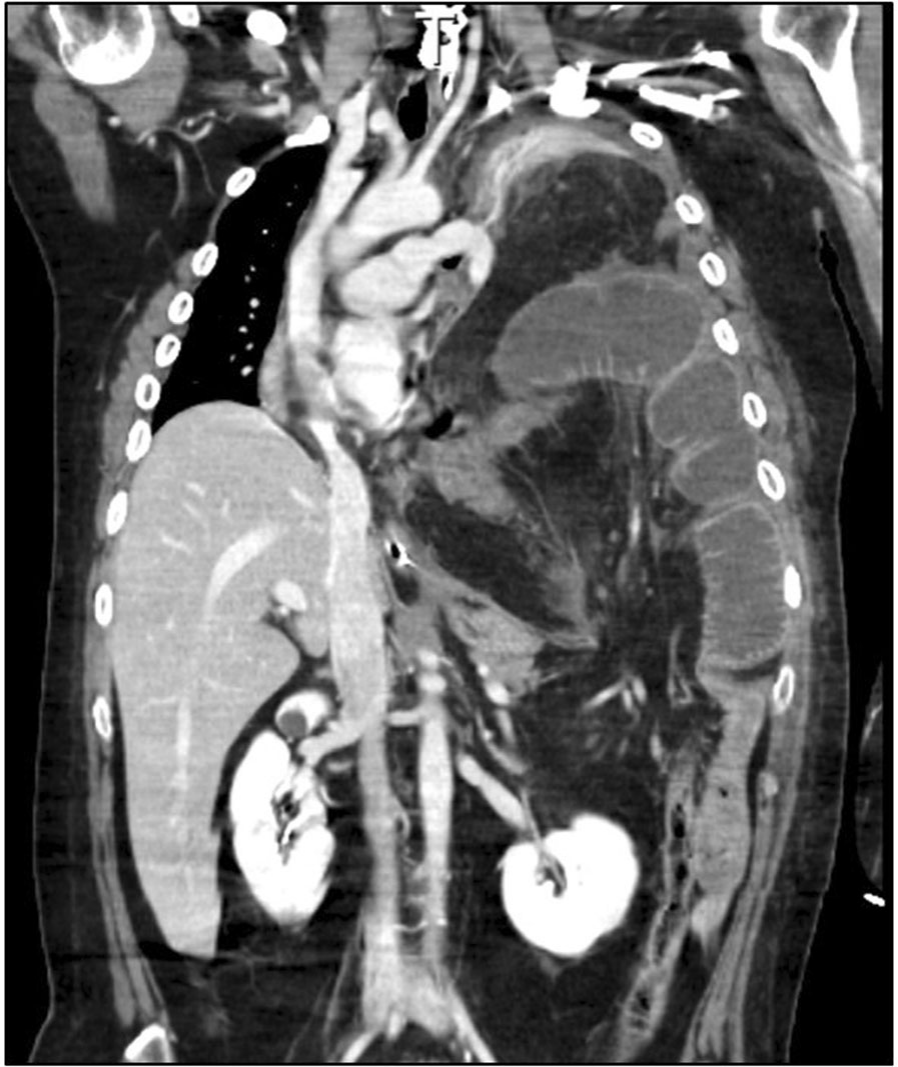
Contrast-enhanced computed tomography (CT). Images demonstrated the presence of a large diaphragmatic hernia, which allowed the small intestine, transverse colon, and omentum to herniate into the left chest. Portions of these herniated organs showed decreased blood flow. Collapse of the left lung and marked mediastinal shift to the right were apparent
